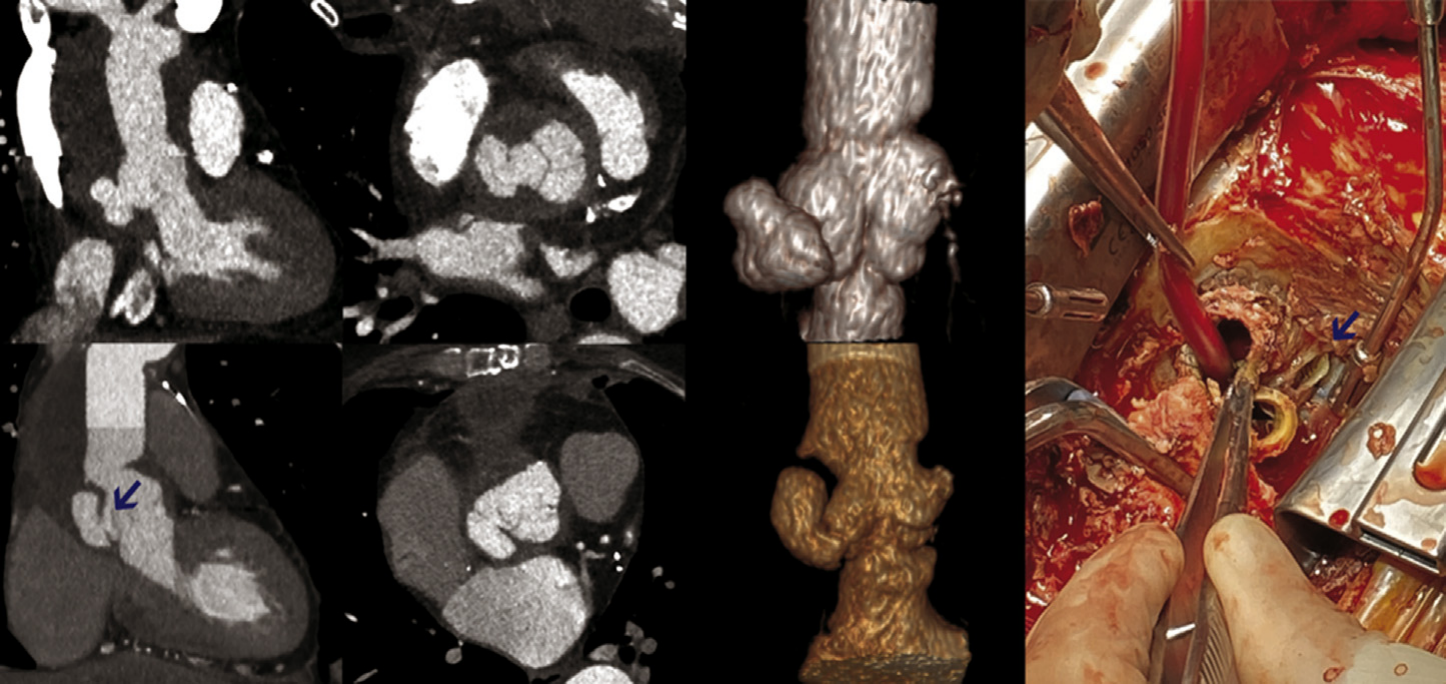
Left*, Computed tomography and 3-dimensional reconstruction (cases 1 and 2); *right*, operative image rupture of the noncoronary sinus (case 2, *arrow*).
